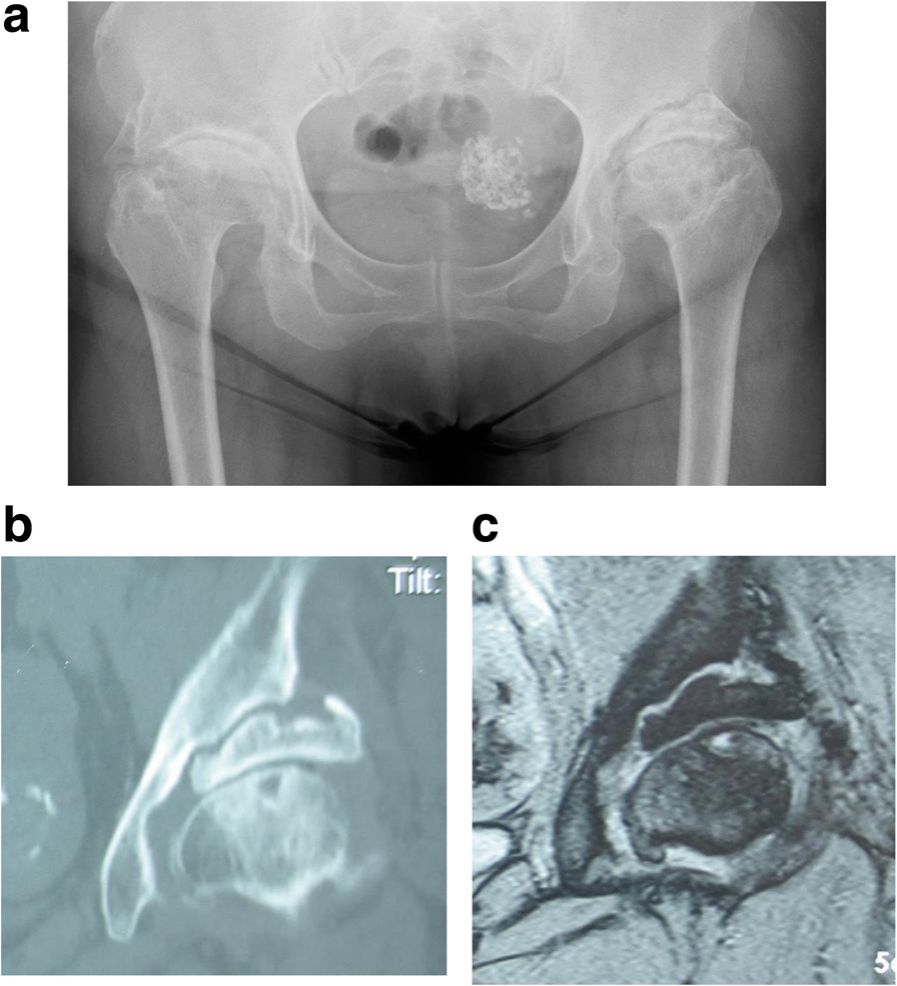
Imaging findings from the first examination. a Plain radiography of the bilateral hip joints reveals no joint space narrowing in the right hip joint. However, joint space narrowing and pseudoarthrosis of the periacetabular osteotomy segment is visible in the left hip joint. b Computed tomography of the bilateral hip joints reveals no bone fusion at the left segment. c Magnetic resonance imaging of the bilateral hip joints reveals pseudoarthrosis at the periacetabular osteotomy mobile bone segment of the left hip joint, with synovial fluid retention. However, no distal segment necrosis is visible
